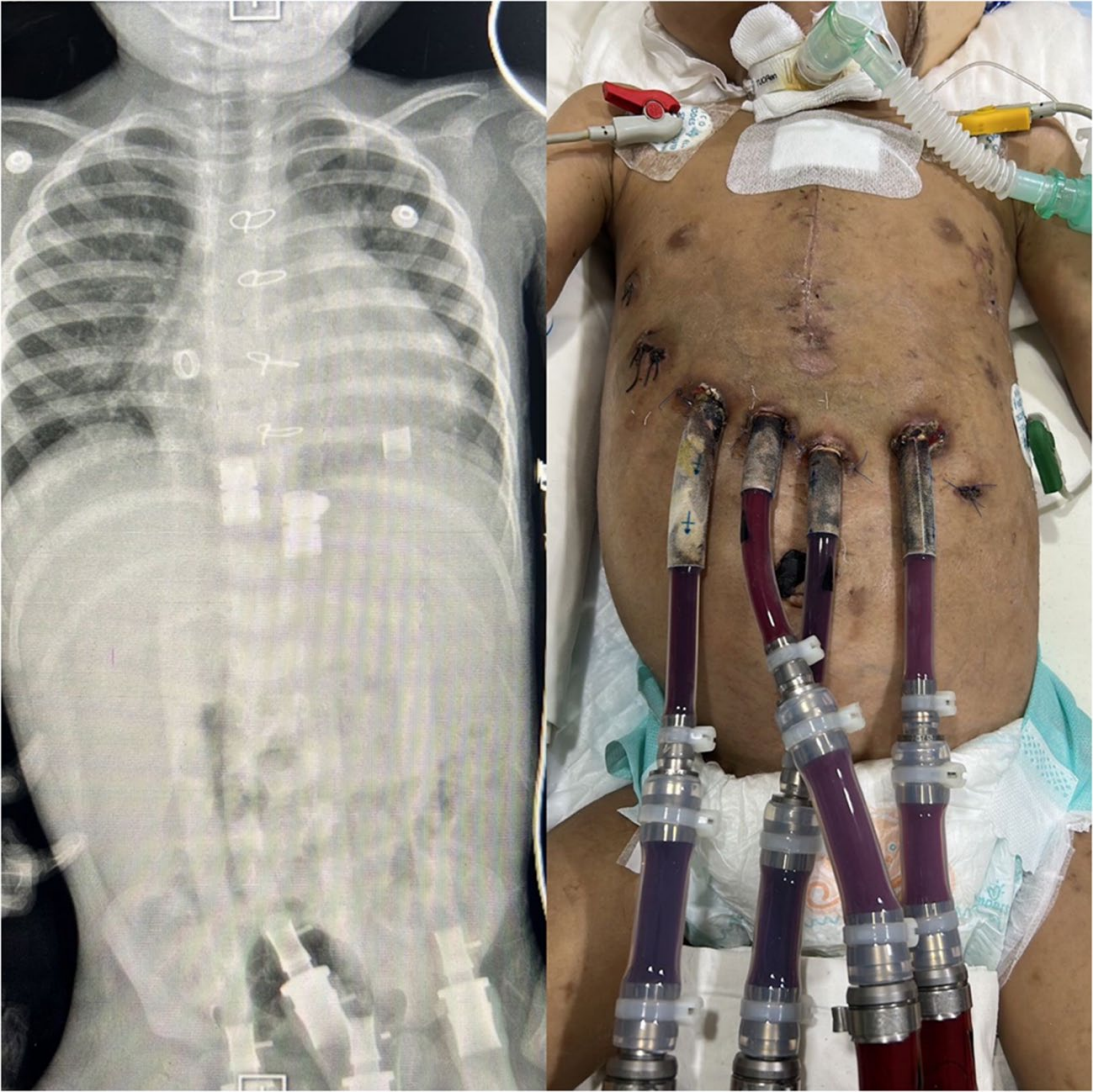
Berlin Heart implantation (with X-ray showing the drivelines)
